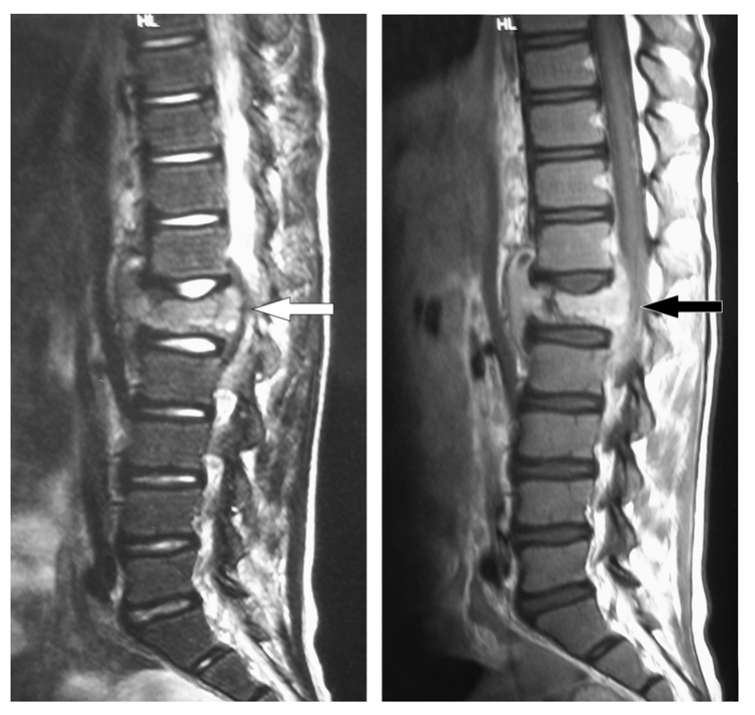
MRI (T1 and T2 weighted images) of lumbar spine. Investigation undertaken 6 weeks after presentation showing loss of height of L1 with surrounding soft tissue mass and impingement on the conus.
